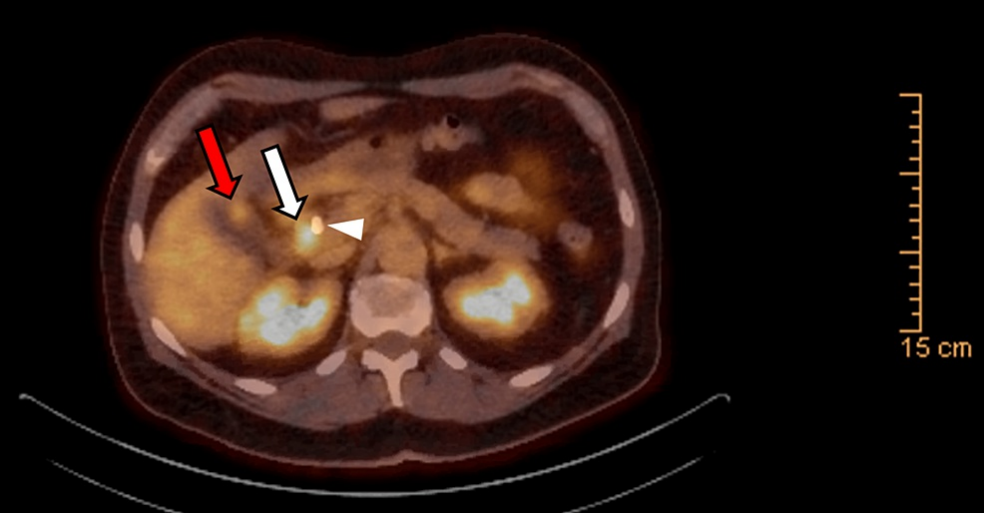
Positron emission tomography demonstrates gallbladder and biliary malignancies There is a 0.9 x 1.4 cm hyperdense lesion in the nondependent portion of the gallbladder (red arrow) with a standardized uptake value (SUV) max of 4.4, suspicious for malignancy. In addition, there is intense fluorodeoxyglucose (FDG) uptake along the biliary stent (arrowhead), SUV max of 7.3, likely representing biliary malignancy (white arrow).
